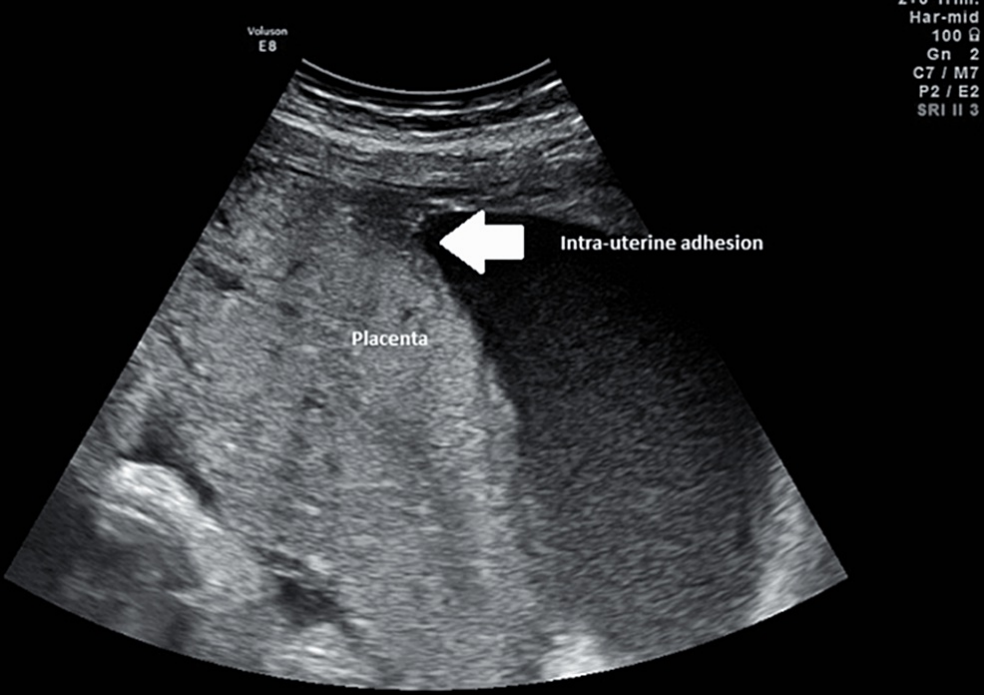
Transabdominal ultrasonography displaying placental implantation on the adhesion (white arrow) and on the posterior uterine wall.
